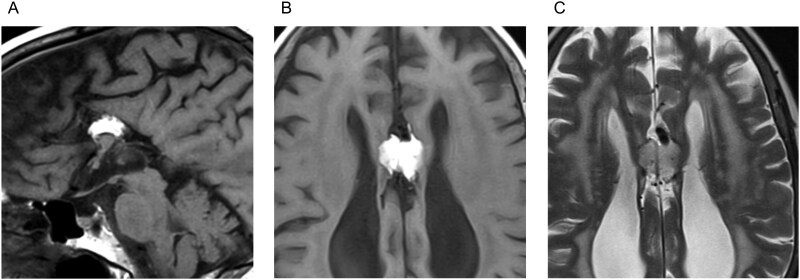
The MRI features of an 85-year-old female with congenital corpus callosum hypogenesis. The medial sagittal plane of the FLAIR image (A) shows hypogenesis of the corpus callosum with lipoma of the corpus callosum. T1-weighted axial images (B) and T2-weighted axial images (C) show colpocephaly and a tubulonodular-type lipoma of the corpus callosum.
